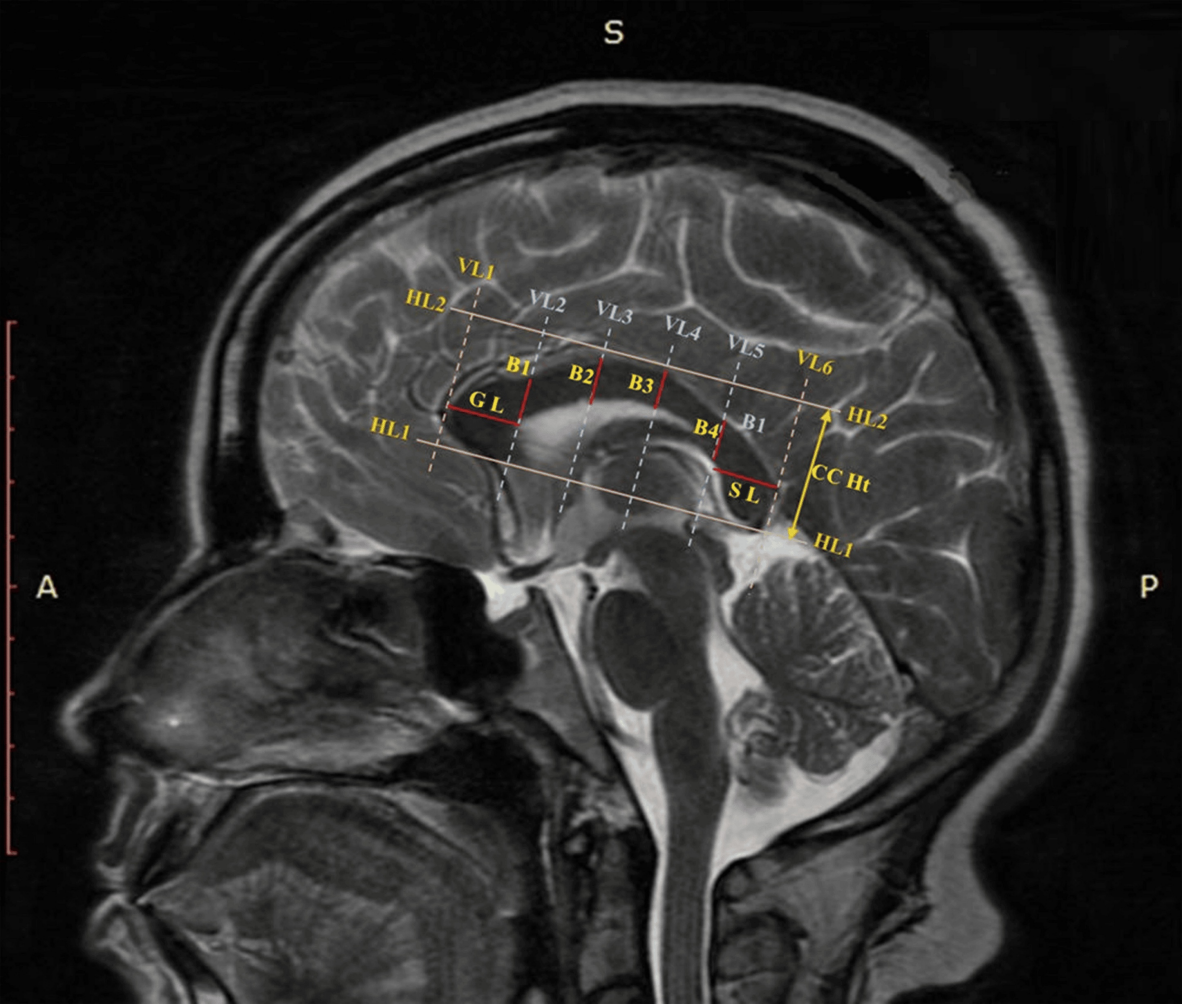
A sagittal T2-weighted MRI of the brain, annotated with multiple linear measurements used in morphometric analysis of the corpus callosum (CC). Reference lines (dashed lines) and the main measurements (solid lines). HL1- Horizontal Line 1 (A tangent connecting the most inferior points of the rostrum and splenium), HL2- Horizontal line 2 (a second reference line following the superior contour of the trunk), VL1- Vertical Line 1 (vertical reference line passing tangentially to the genu and perpendicular to HL1 and HL2),VL2- Vertical Line 6 (vertical reference line passing tangentially to the splenium and perpendicular to HL1 and HL2), Four additional vertical lines VL2- Vertical Line 2, VL3- Vertical Line 3 , VL4- Vertical Line 4, and VL5- Vertical Line 5  which divide the CC into five equal pieces, GL- genu length, B1 to B4- thickness of the first part, second, third and fourth parts of the body of CC, SL – length of the splenium of CC, CC Ht- height of CC (the distance between HL1 and HL2). A modified version of the Witelson Partitioning (WP) scheme [25] was used for taking various measurements.
